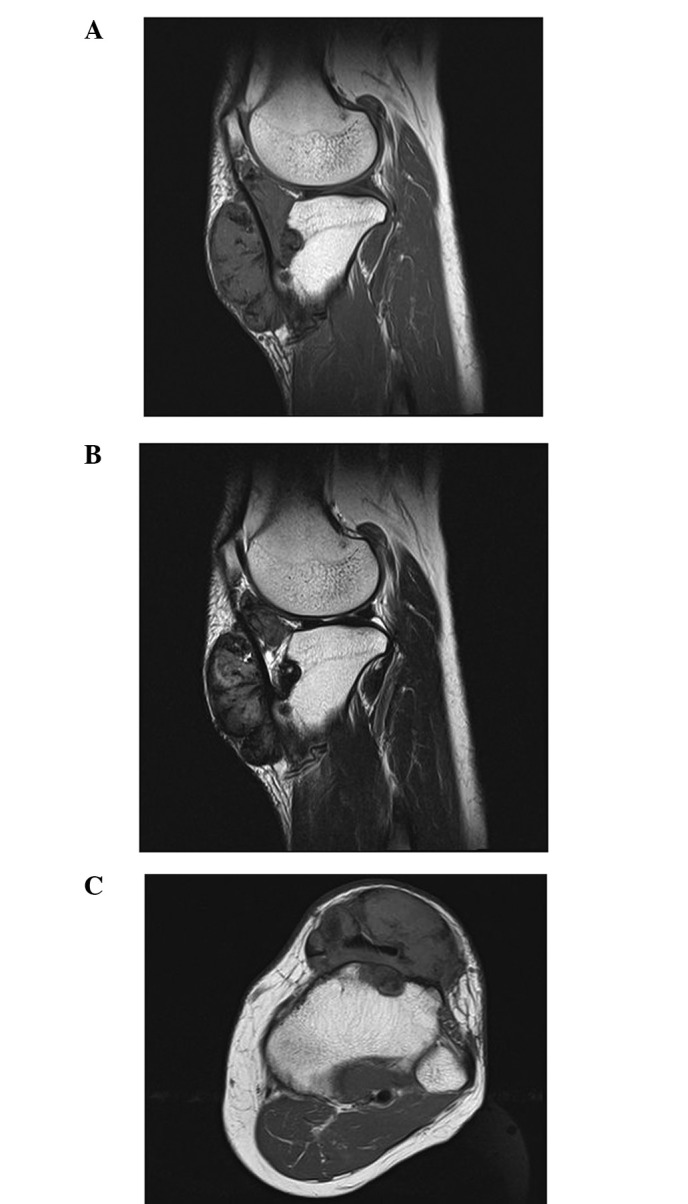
Sagittal magnetic resonance images of the left knee. (A) T1-weighted (repetition time/echo time [TR/TE]=540/12 msec) and (B) T2-weighted (TR/TE=4000/84 msec) images demonstrate a well-circumscribed soft tissue mass in the infrapatellar fat pad posterior to the patella tendon. (C) The axial image reveals a tumor wrapped around the entire circumference of the patellar tendon.
